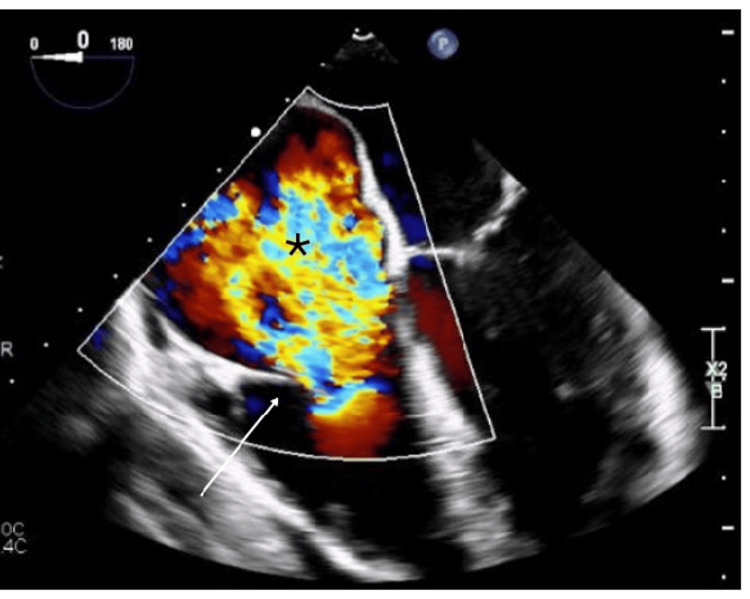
Transoesophageal echocardiography showing mild right atrial dilatation (*), tricuspid annular dilatation with severe tricuspid regurgitation (arrow) (effective regurgitant orifice area of 0.7 cm², regurgitant volume of 57 mL) and preserved biventricular function.
